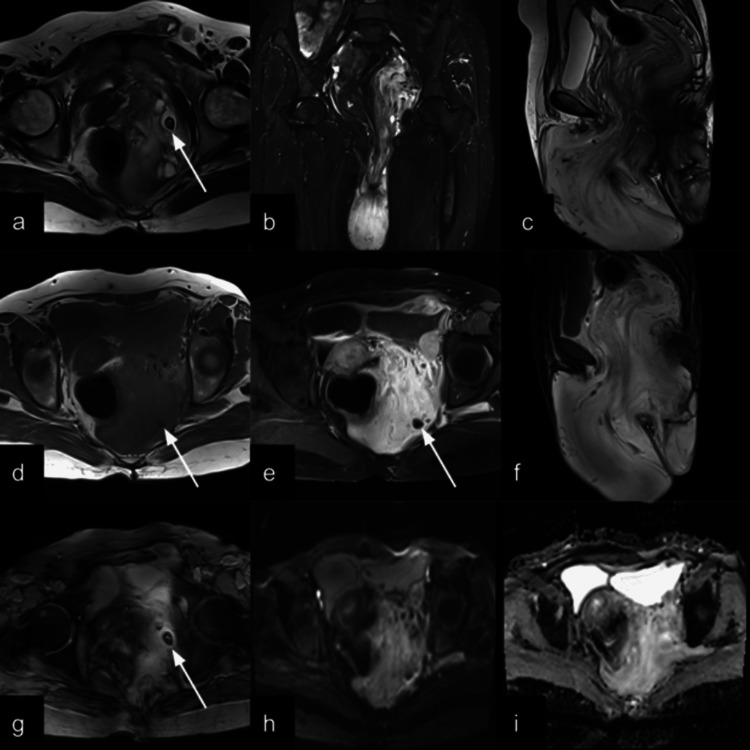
T2-weighted axial (a), STIR coronal (b), and T2-weighted sagittal (c) images show a T2 hyperintense mass with laminated morphology extending from the pelvis into the perineum. Sagittal T2 (c) shows the longitudinal extent of the mass. On the T1-weighted image (d), the mass is isointense. Post-contrast T1 fat-saturated images in axial (e) and sagittal planes (f) showing intense contrast enhancement of the mass. DWI images with B value 800 (h) and the ADC map (i) showing facilitated diffusion (mean ADC value 2.165 × 10⁻³ mm²/s). Axial (a), coronal (b), and sagittal (c) T2-weighted images of the patient show a hyperintense mass with a swirled appearance in the pelvis herniating into the perineum, displacing the hollow pelvic structures. White arrows showing calcification in T2 (a), T1 (d), T1 post-contrast (e), and T2-gradient echo images (g). STIR: short tau inversion recovery; DWI: diffusion-weighted imaging; ADC: apparent diffusion coefficient
